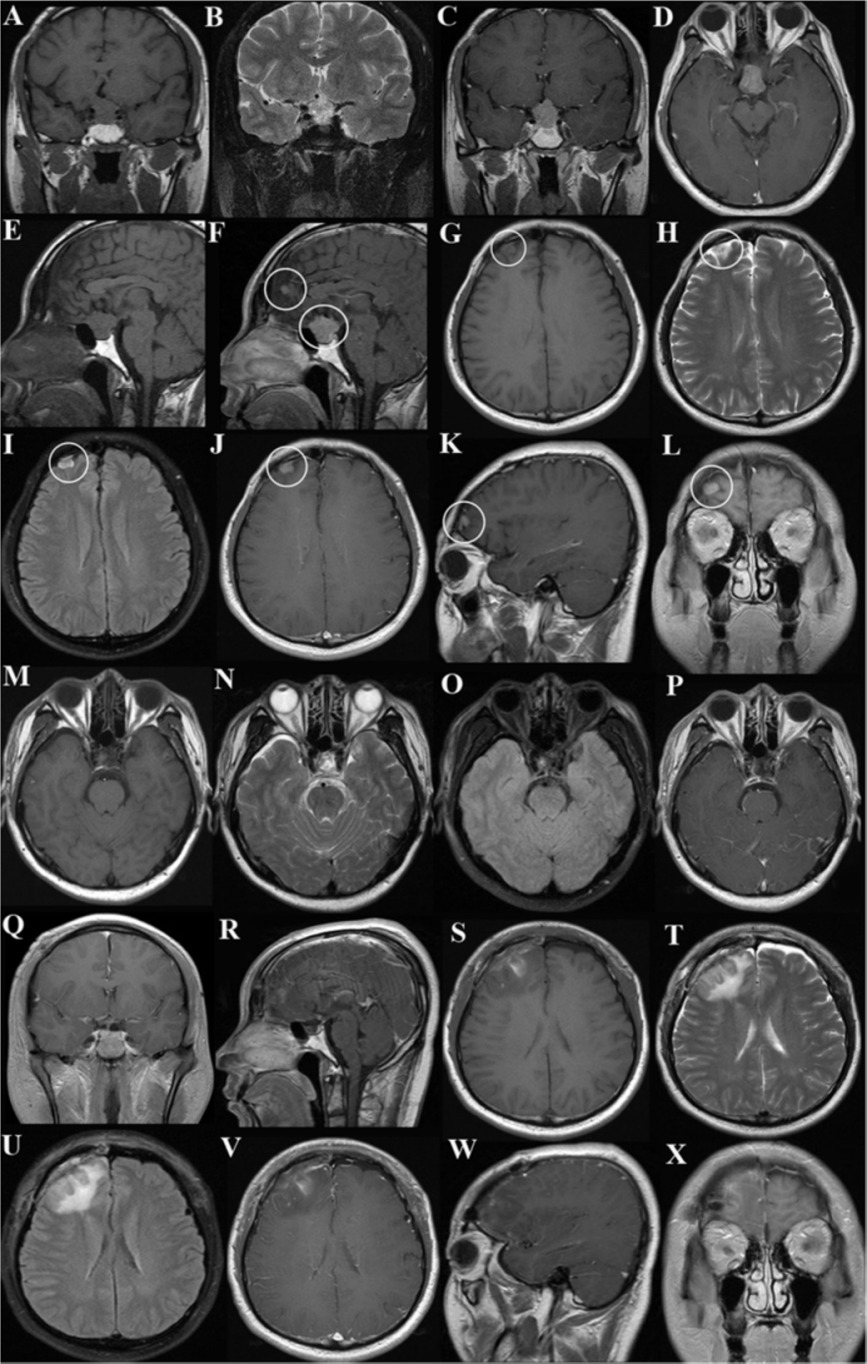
Pre- and postoperative magnetic resonance imaging scans from the fourth surgery.
